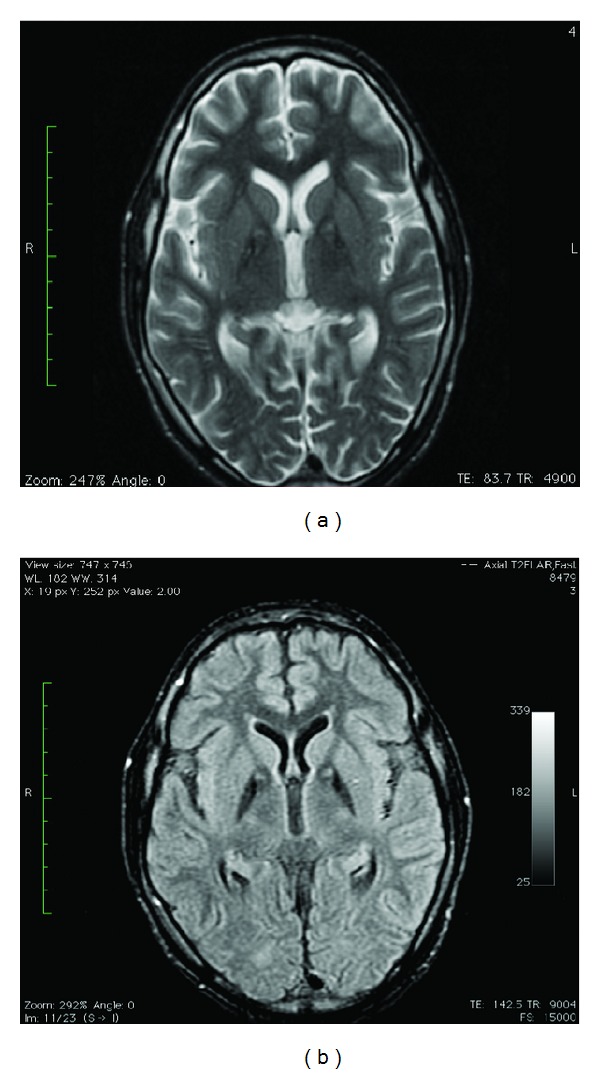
(a) Axial T2W fse. (b) Axial T2 FLAIR. Both demonstrate T2 shortening (hypointensity) with foci of T2 prolongation (hyperintensity) at the anteromedial aspect in the bilateral globus pallidus (eye-of-the-tiger sign).
